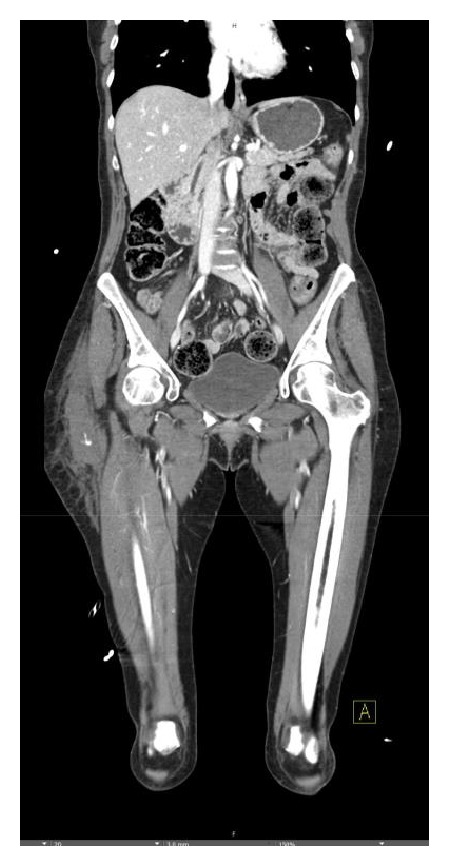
Coronal CT reconstructed images further detail the area of active extravasation and surrounding hematoma.
